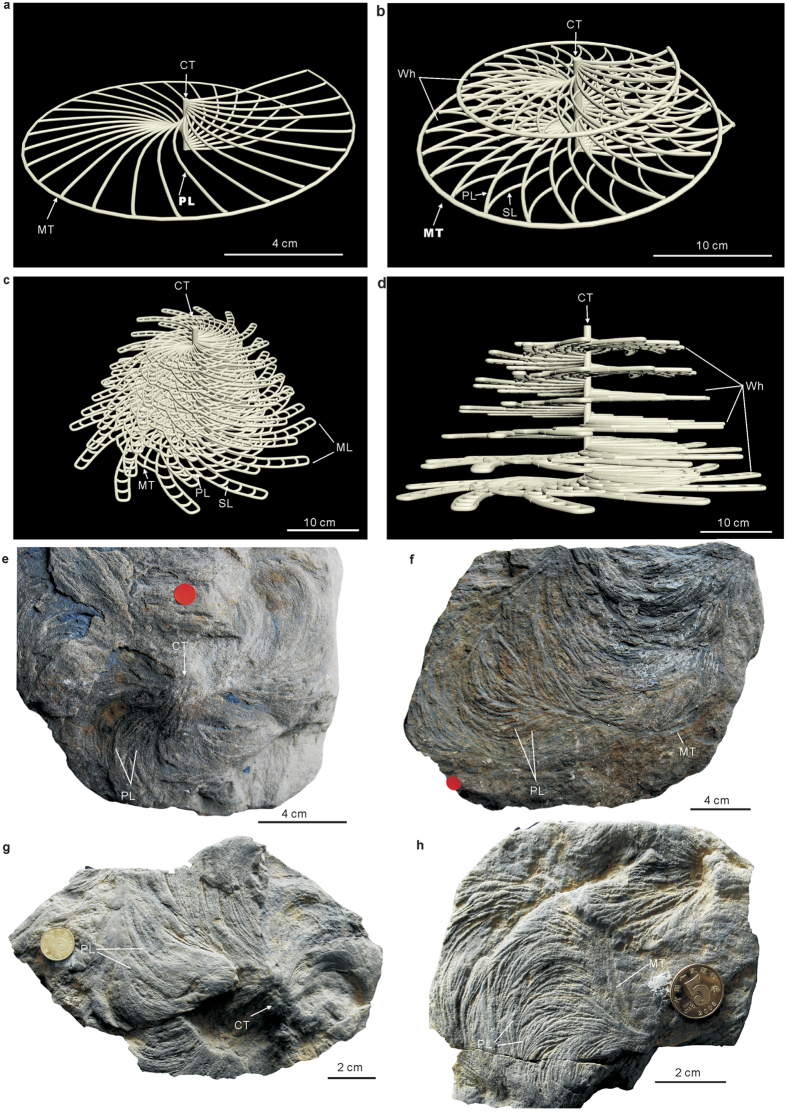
Morphology of *Zoophycos*. (a–d) Three-dimensional model of *Zoophycos*, including protrusive and retrusive spreiten, but here we just show the protrusive ones. (a) Palaeozoic *Zoophycos*. Simple, helicoidal circular spreiten with one whorl. (b) Mesozoic *Zoophycos*. Simple–complex, helicoidal circular spreiten with two–five whorls. (c,d) Cenozoic *Zoophycos*. Complex, helicoidal lobate spreiten with several whorls. (e–h) Examples of Devonian *Zoophycos*, helically coiled circular (e,f) or tougue-like (g,h) spreiten, with distinctive primary lamellae, marginal tube and cylindrical tunnel, secondary lamellae not well preserved. (e,f) *Zoophycos* from the Givetian Songjiaqiao Fm., Dushan, Guizhou, South China, upper bedding surface. (g,h) *Zoophycos* from the Givetian Tiaomajian Fm., Guanyang, Guangxi, South China, upper bedding surface. Abbreviation: CT = cylindrical tunnel, MT = marginal tube, PL = primary lamellae, SL = secondary lamellae, ML = marginal lobes, and Wh = whorls. (a–d) were designed and made by Li-Jun Zhang using the 3DMAX software, (e–h) were collected by Li-Jun Zhang and Yi-Ming Gong in the field and taken photos in the Paleontology Lab of State Key Laboratory of Biogeology and Environmental Geology by Li-Jun Zhang.
